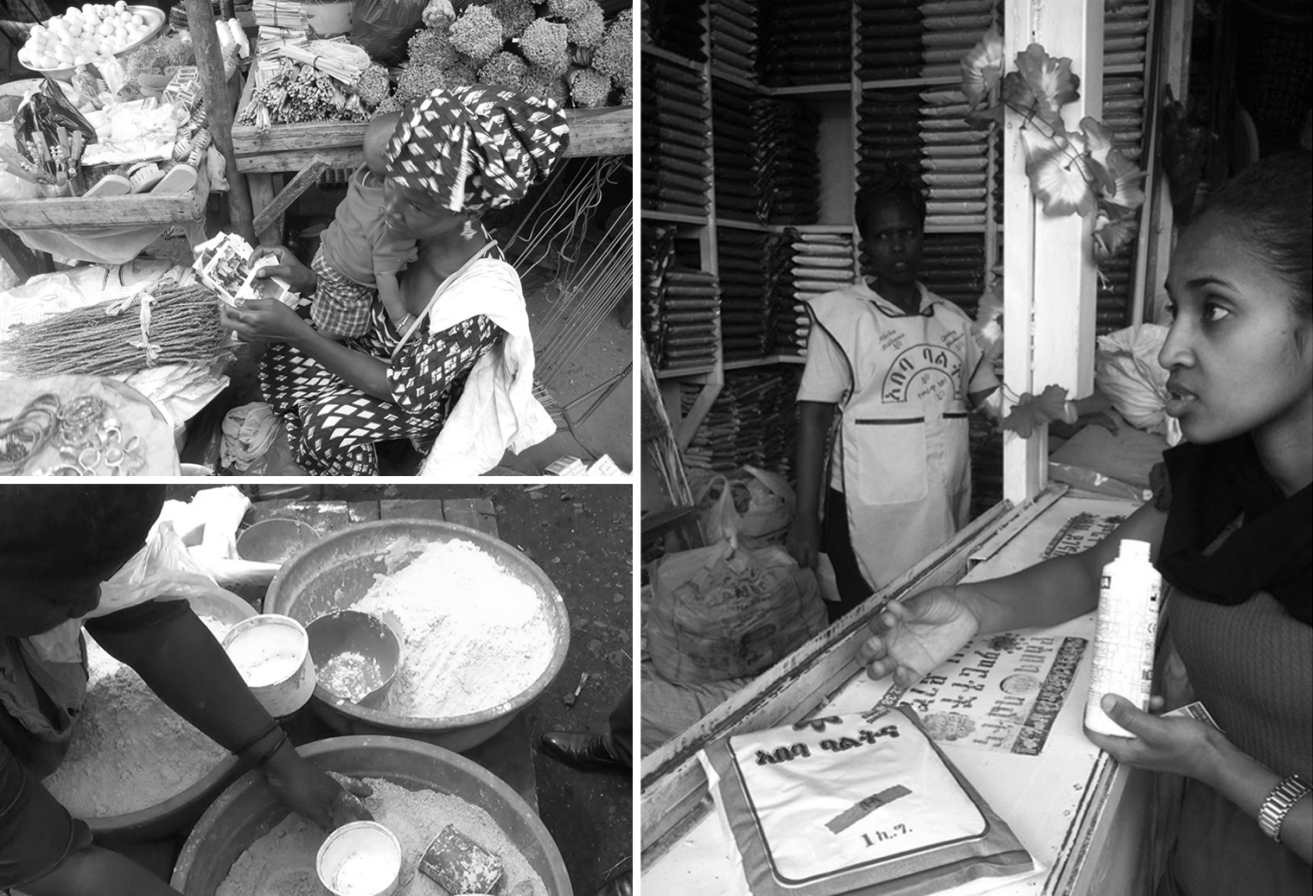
Markets in Goma, Addis Ababa, and Bameko. (A) Vendors are interviewed in Addis Ababa Ethiopia at Merkato in Lideta sub city and Addis Ketema by research colleague Alegnta Gebreyesus, MD in Amharik. (B) Vendors are interviewed at the Virunga Market in Goma, DRC by research assistant, Rene Zaidi, in Kiswahili. Commercial ultrasound gel is demonstrated and this vendor suggests banana flour, sorgum or cassava root flour. (C) Vendors are interviewed at Bameko Mali by research assistant, Bouba, in Bambera. The vendor reviews photos of obstetric ultrasounds and suggests banana flour, sorgum or cassava root flour.
